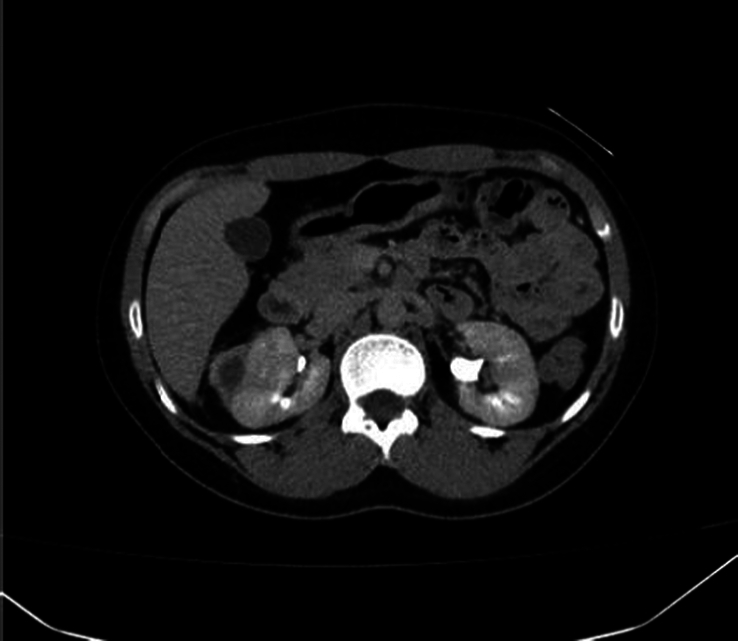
Computed tomography scan showing great regression in the subcapsular hematoma on the right kidney.
